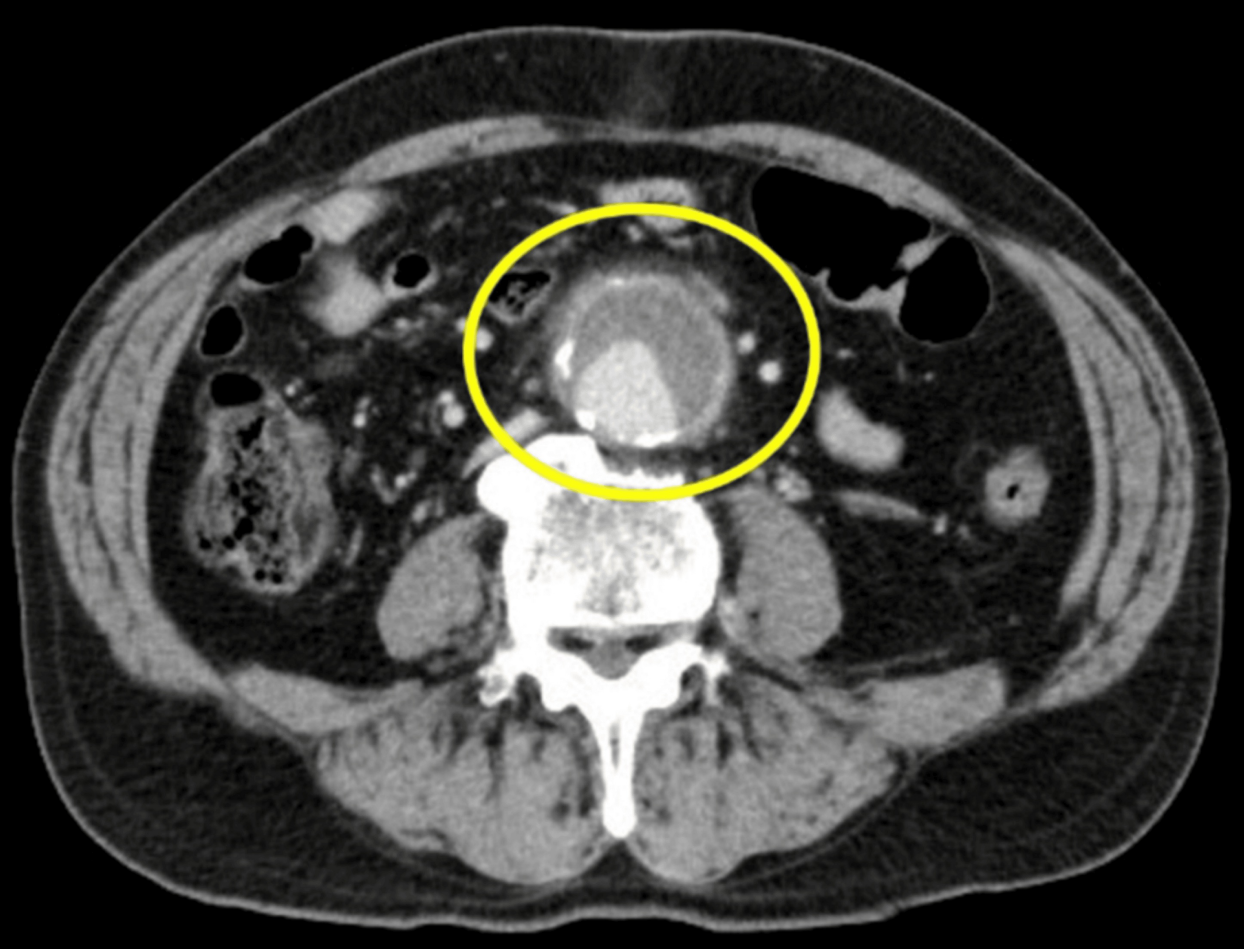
Contrast-enhanced CT of the abdomen and pelvis on admission The scan shows a 48-mm infrarenal abdominal aortic aneurysm (yellow circle) with increased attenuation of the surrounding adipose tissue extending from the anterior to the lateral aspect of the infrarenal aorta.
